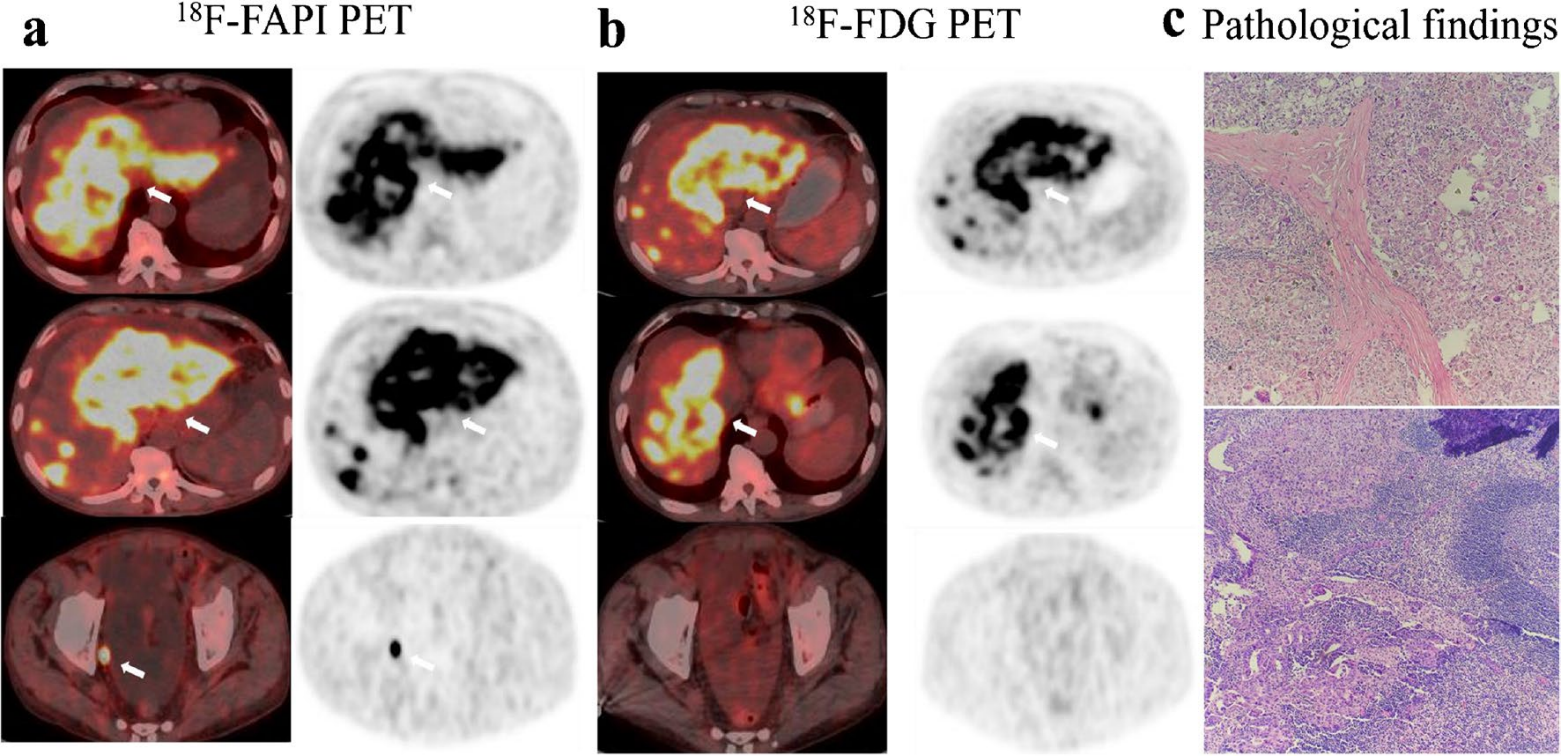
A 49-year-old male underwent PET/CT to evaluate a liver mass previously detected through ultrasound. a, b Intense metabolic activity was observed on ^18^F-FAPI-04 PET/CT images in the both primary lesion (SUVmax = 9.7) and lymph node (SUVmax = 3.3) (arrows). c, d The paired ^18^F-FDG PET/CT images also showed obvious uptake in the primary tumor (SUVmax = 6.9) but no uptake in the regional lymph node. e The pathological results derived from a liver mass revealed a primary poorly differentiated hepatocellular carcinoma. The pathological findings derived from lymph node resection are indicative of metastatic one
